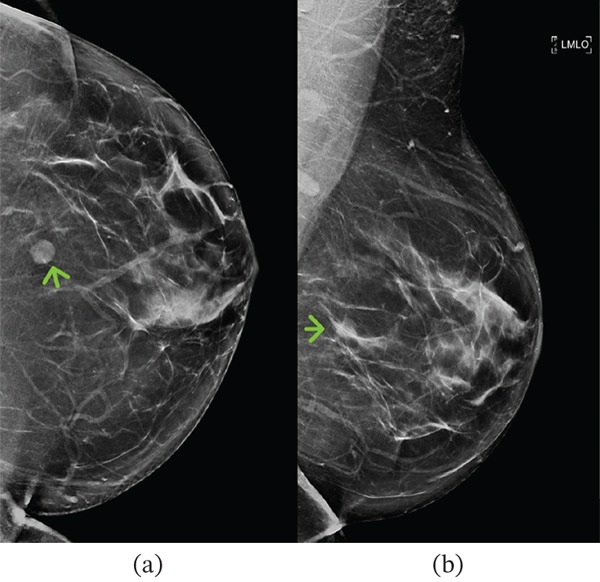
3D tomosynthesis mammogram of the left breast in (a) CC and (b) MLO projections demonstrates a circumscribed, round, 9‐mm mass (green arrow) deep in the breast, slightly lateral to the posterior nipple line. BI‐RADS 0 (incomplete; ultrasound recommended).
